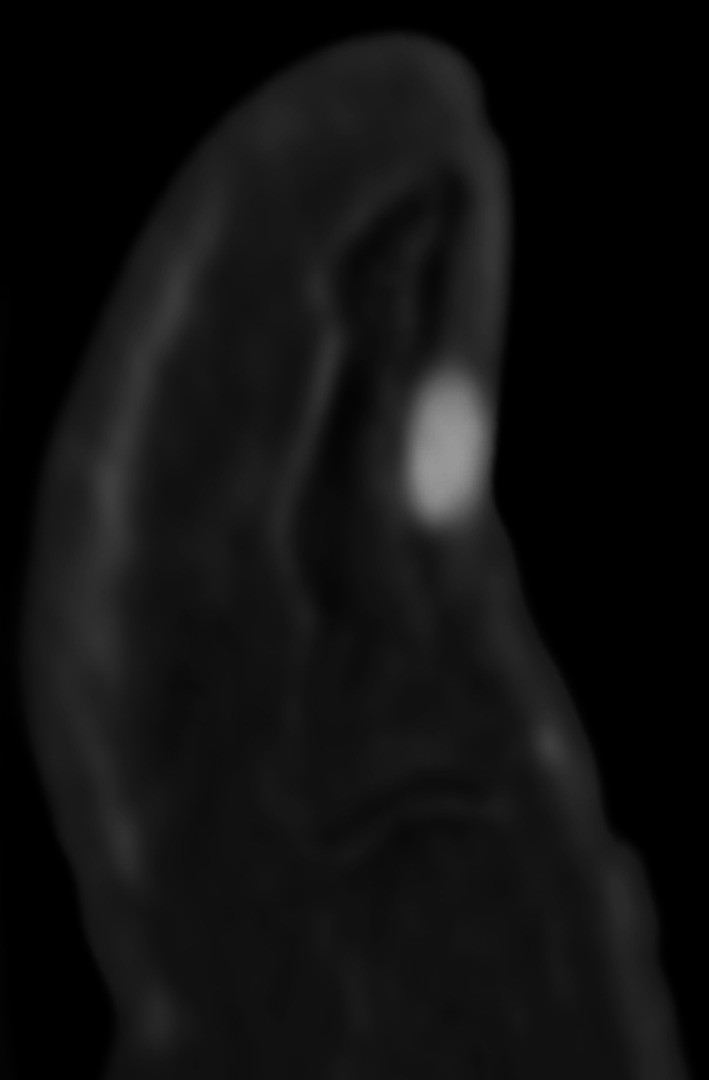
Glomus tumour of the thumb in a 68-year-old man. Dynamic CTA with bone subtraction showing a small hypervascularised tumour (arrow) of the nail bed
